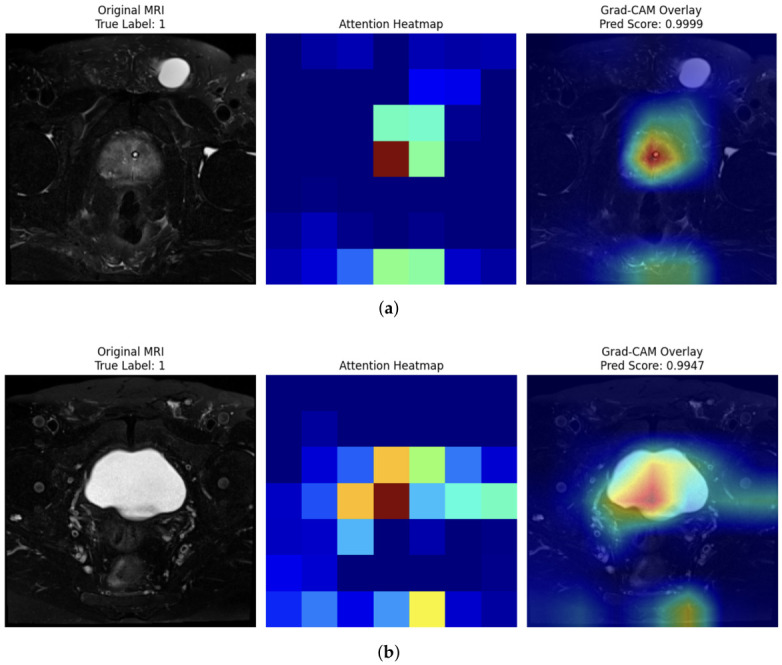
Grad-CAM explainability analysis on T2-weighted MRI test samples. (a) The model correctly classifies a clinically significant lesion (True Label: 1) with a near-perfect prediction score of 0.9999. (b) Another positive case (True Label: 1) is correctly identified with a prediction score of 0.9947.
